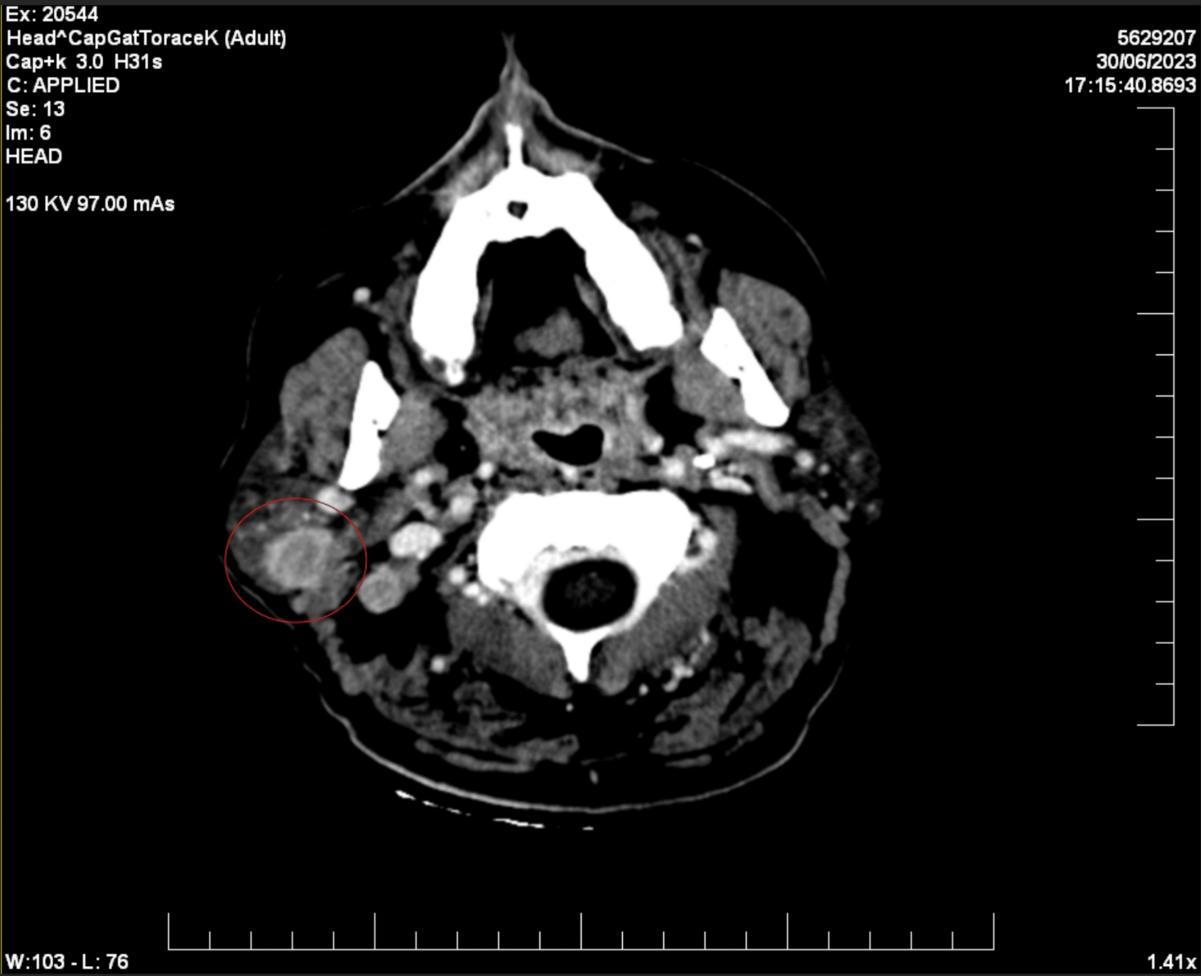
CECT showing a nodular mass within the right parotid gland (red circle). CECT: contrast-enhanced computed tomography
